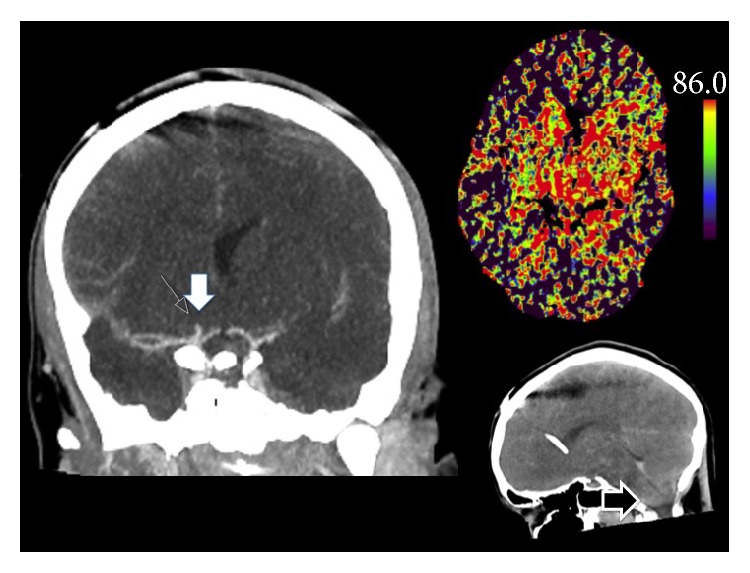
Second computed tomography of the head (02:30 am) shows an aneurysm at the right carotid T cross, pan-cerebral diminished perfusion, and developing tonsillar herniation.
